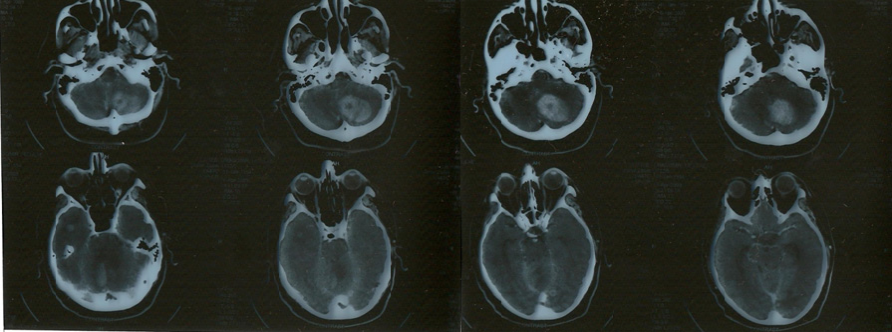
The brain contrast CT scan performed 24 hours after
